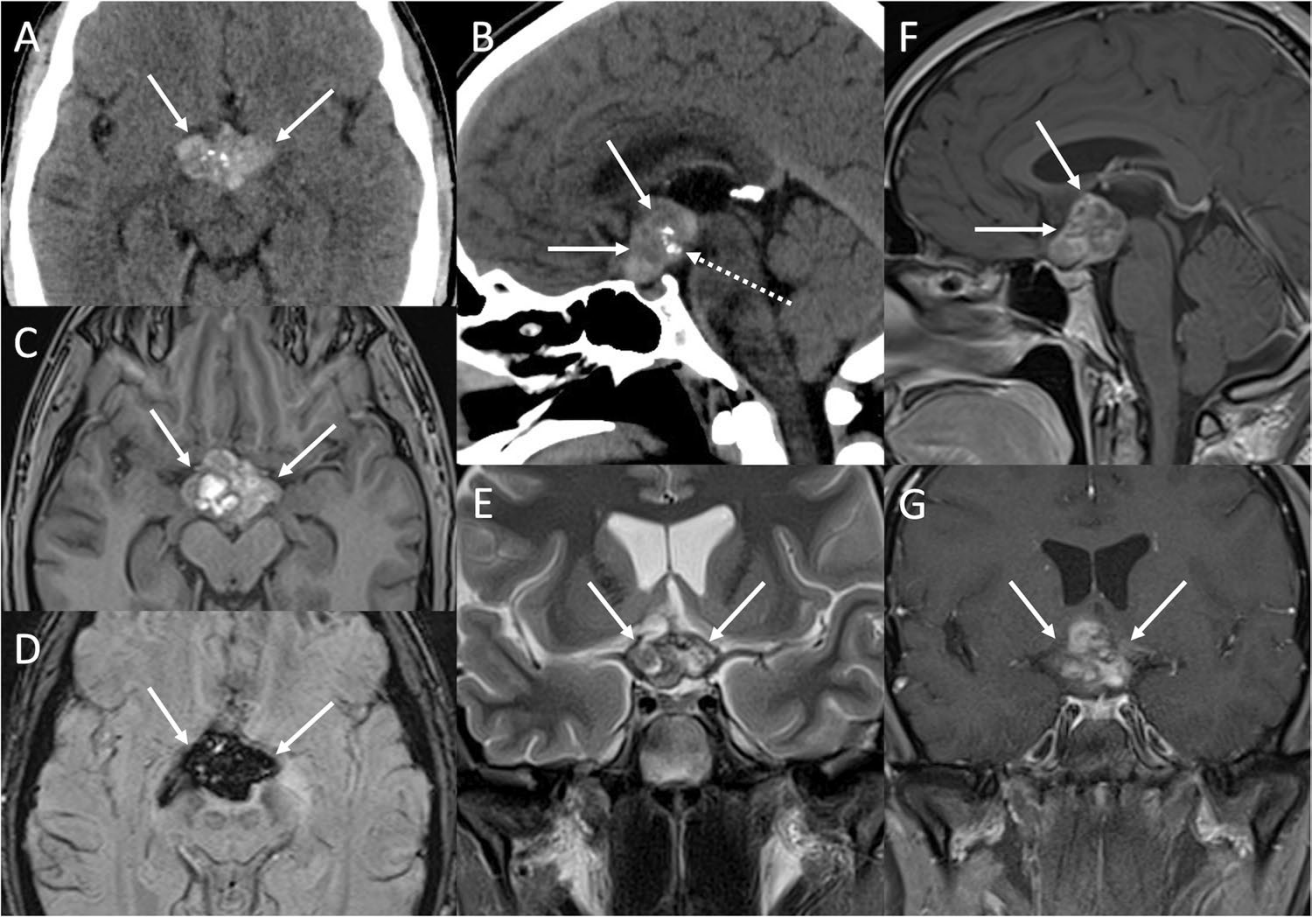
Chiasmatic cavernous venous malformation. Axial (A) and sagittal (B) CT reformats show a suprasellar multilobulated mass (arrows), slightly hyperdense with small dystrophic intralesional calcifications (dotted arrow). The lesion presents high signal on T1- (C), low signal on SWI (D), and appears inhomogeneous on T2-weighted (E) MR images. Mild capsular enhancement is evident on sagittal (F) and coronal (G) contrast-enhanced T1-weighted sequences
